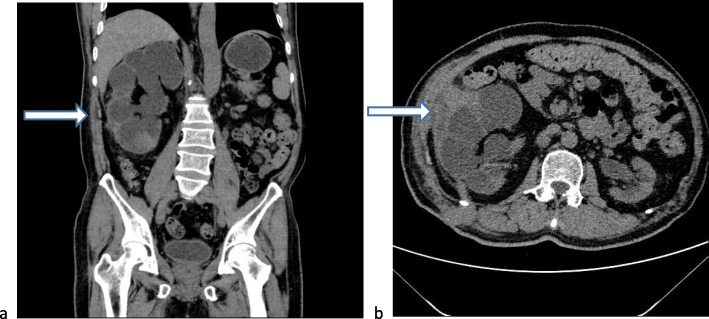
Coronal computed tomography (CT) (a) and axial CT (b) showing a hydronephrotic right kidney partially adhering to the flank. *White arrow* shows the skin involvement site
